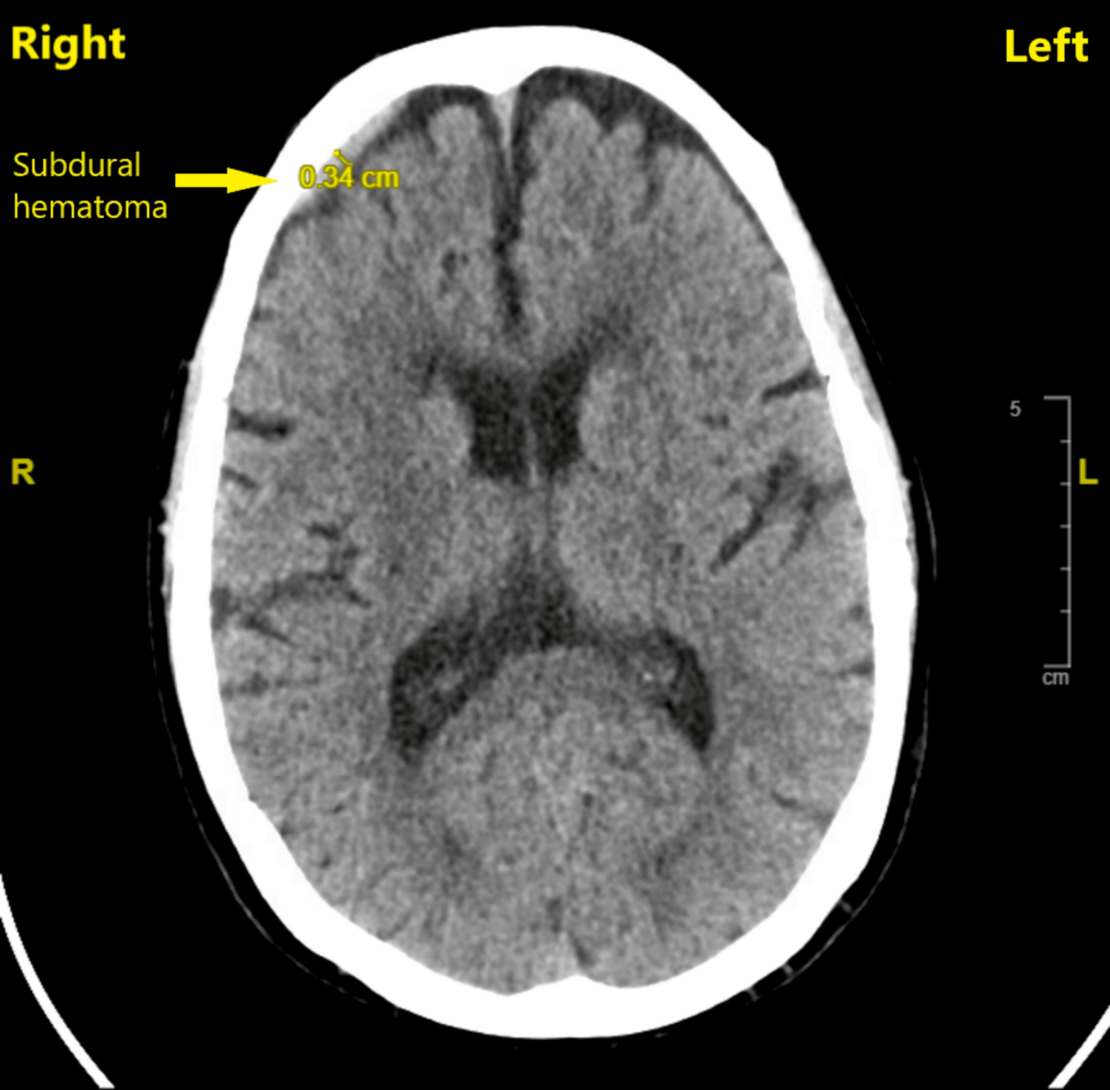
Computed tomography of the head with subdural hematoma overlying the right frontal lobe without any evidence of midline shift Computed tomography of the head performed at the initial evaluation of headache that the patient presented with five days after spinal anesthesia, revealing a subdural hematoma in the right frontal lobe without any midline shift and mass effect.
